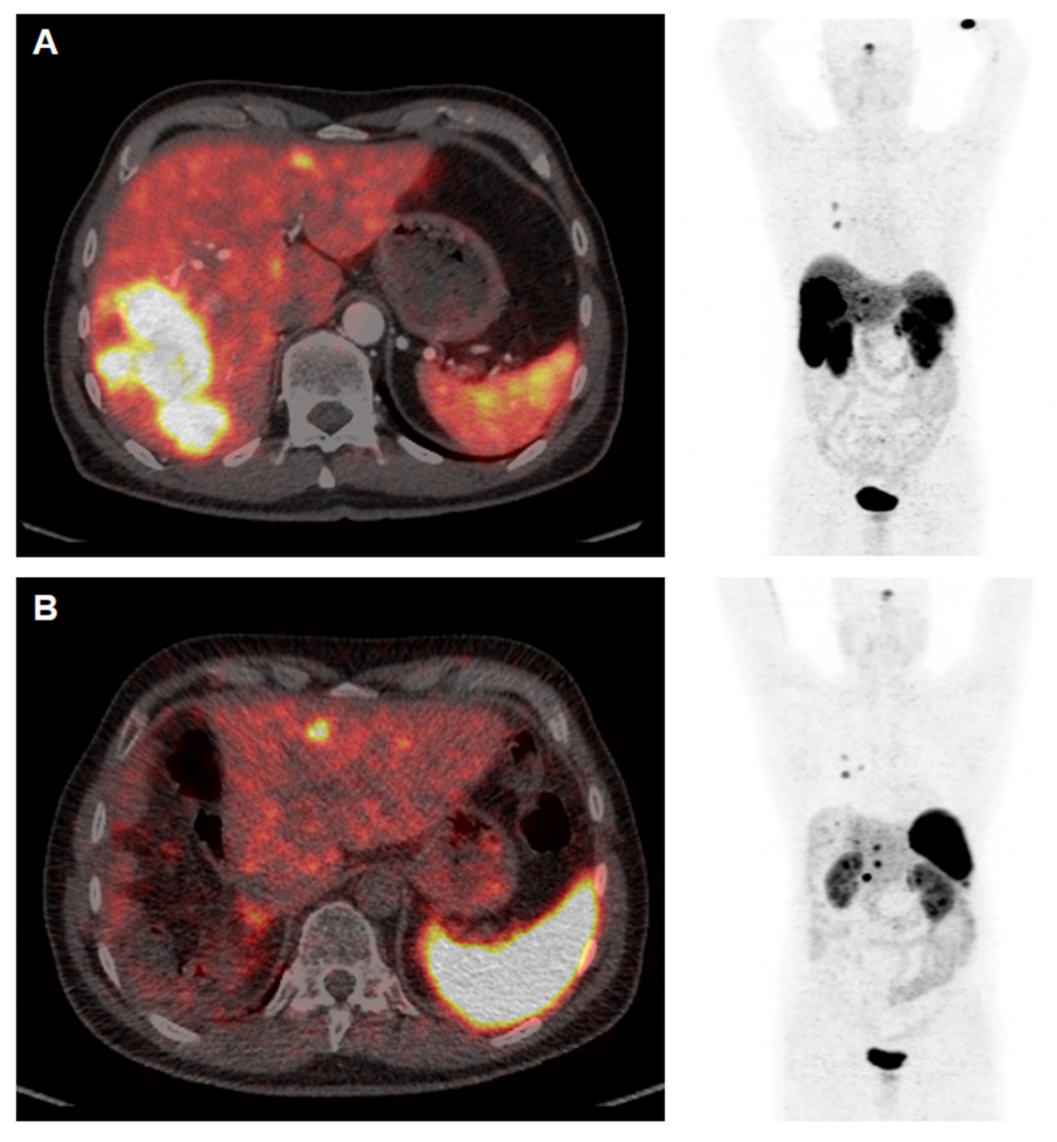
[^68^Ga]Ga-DOTATOC PET/CT Study Prior to (A) and 12 weeks after (B) Cytoreductive Surgery. The patient (64 y) was diagnosed with medullary thyroid cancer (MTC) at stage pT1a N1a R0 Mx in 2005. After complete thyroidectomy in July 2005 the disease was stable until 2014 when SSTR-positive liver metastases were diagnosed. PRRT with [^177^Lu]Lu-DOTATATE (accumulated activity 29.47 GBq) then resulted in disease stabilisation until January 2022. Appearance of increased size of liver metastases (A) were associated with increased episodes of watery diarrhea, not responsive to symptomatic therapy. In April 2023 the patient underwent cytoreductive surgery (B) and currently receives a postsurgery second period of [^177^Lu]Lu-DOTATATE PRRT. Symptoms are completely relieved and the patient has gained >10 kg body weight (September 2023).
